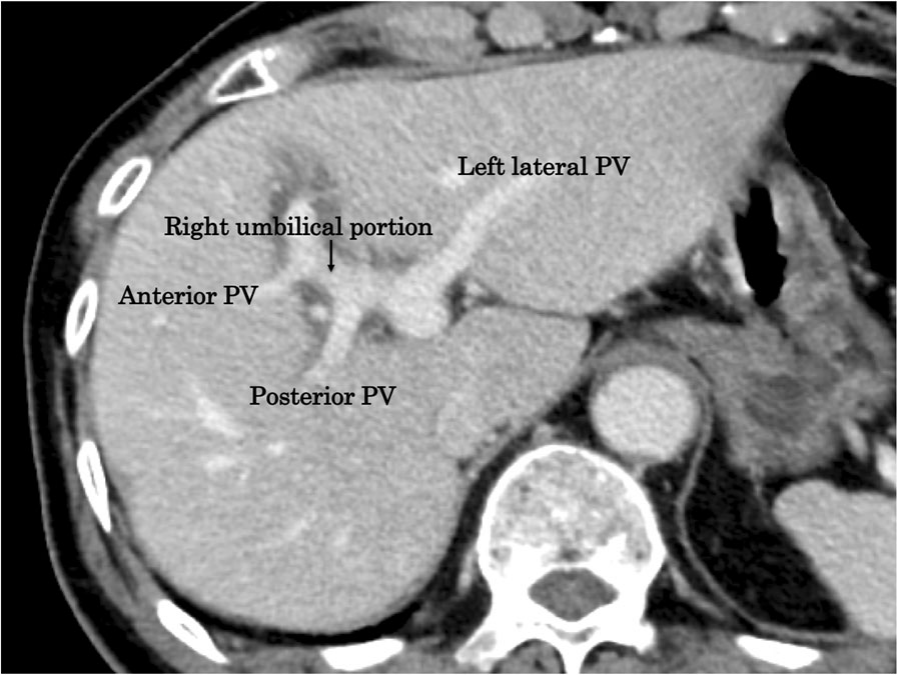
Abdominal contrast-enhanced computed tomography findings. The intrahepatic portal vein formed the right umbilical portion (black arrow)
